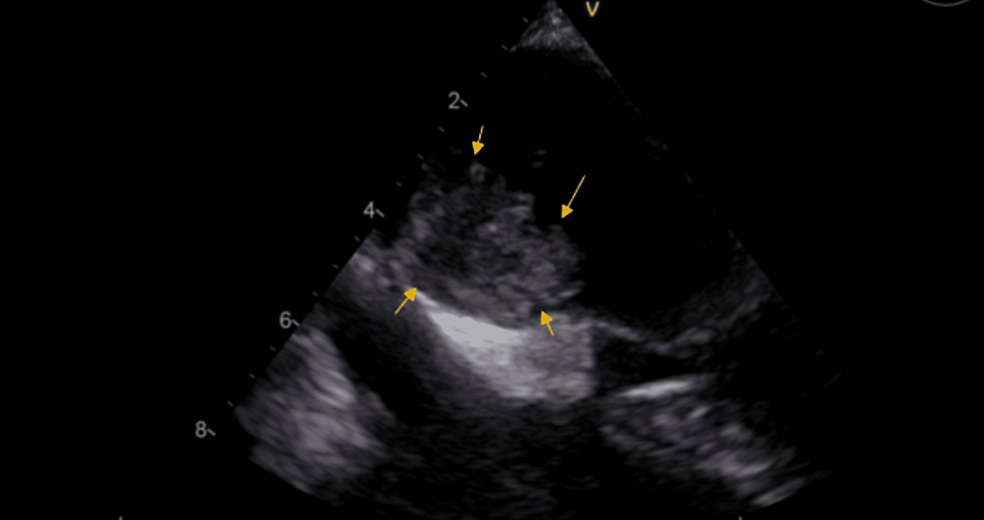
Transthoracic echocardiography showing a 2 cm X 2.1 cm mobile mass in the left atrium
